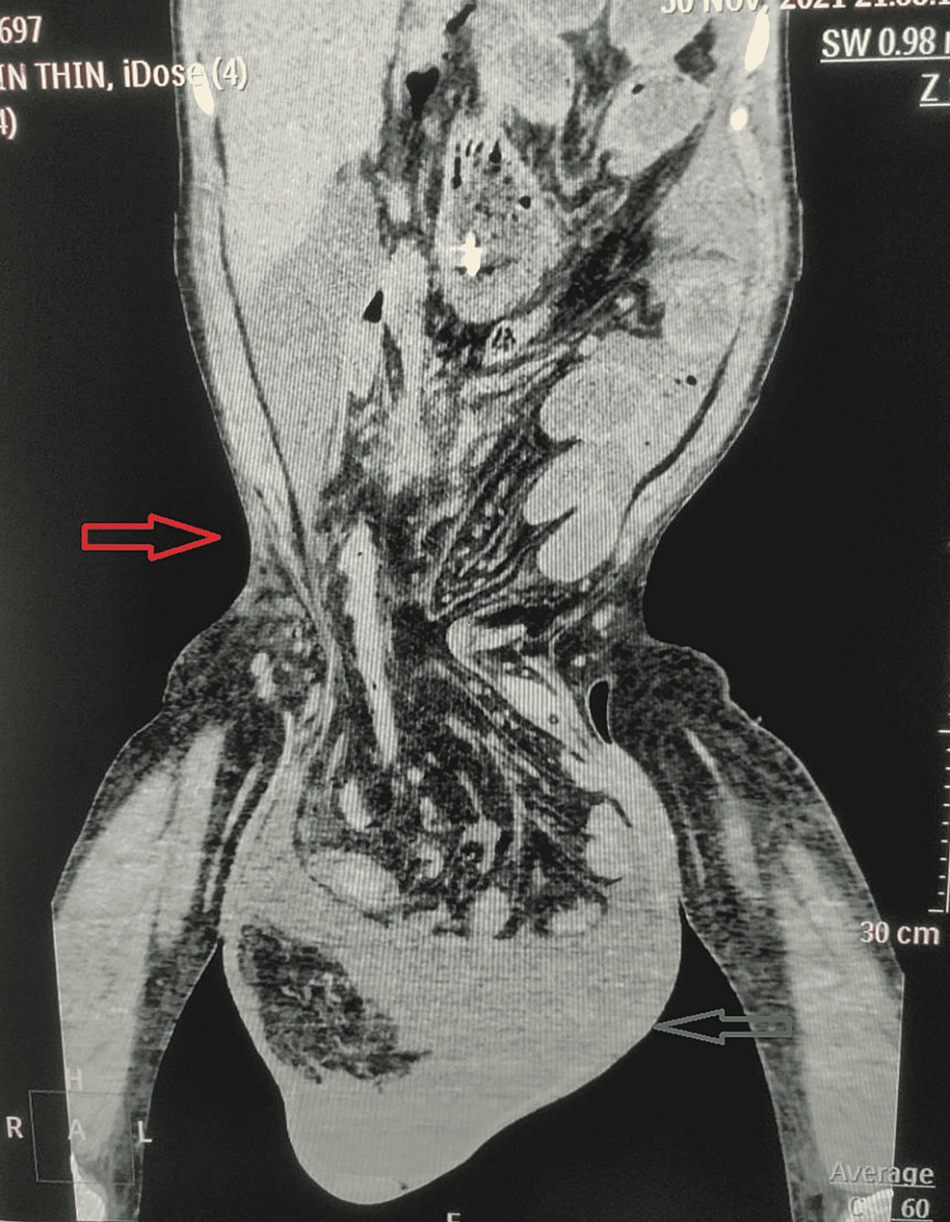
Noncontrast CT coronal view depicting herniation of the abdominal contents into the scrotum. CT: computed tomography
